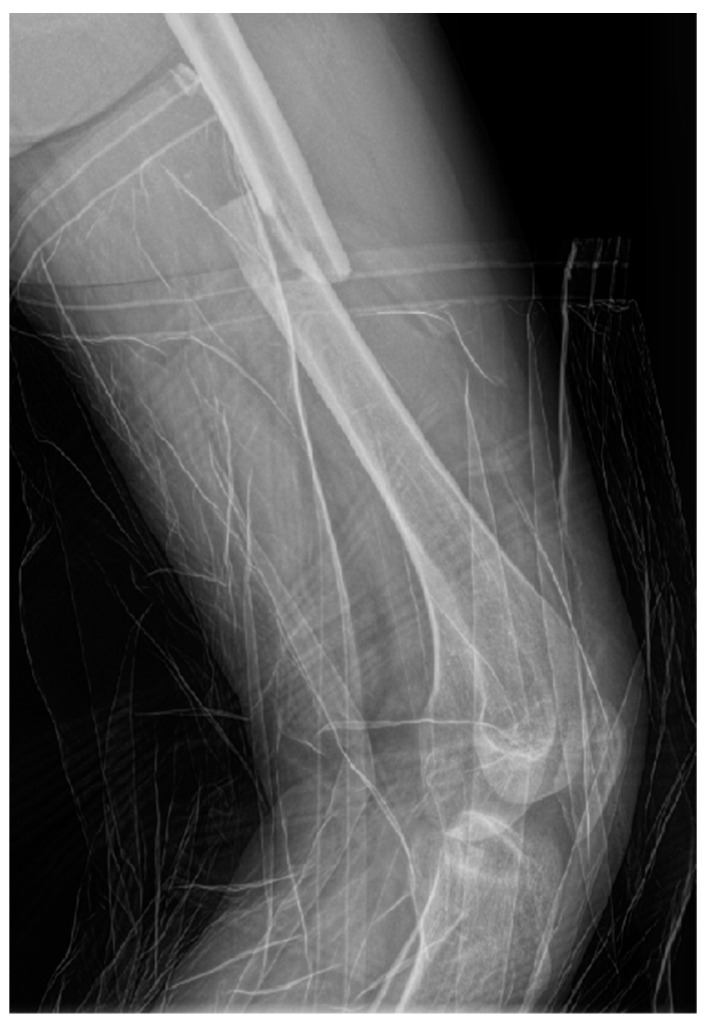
Profile X-ray of the left thigh revealing a fracture of the middle third of the femur with translation of the fragments.
